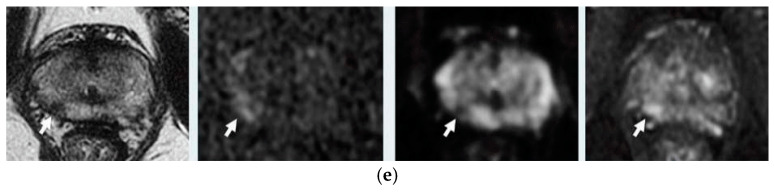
Axial MRI example in post-radiation therapy (RT) by Pecoraro et al. [16]. (a) MR images in 82-year-old man with biochemical recurrence (BCR) (prostate-specific antigen–PSA = 0.09 ng/mL) without suspicious foci of local recurrence, Prostate Imaging for Recurrence Reporting PI-RR 1. (b) MR images in an 85-year-old man with BCR (PSA = 0.09 ng/mL), show diffuse hyperintensity ta high-b value diffusion-weighted imaging DWI (arrows), PI-RR 2. (c) MR images in a 68-year-old man with BCR (PSA = 5.2 ng/mL) indicate hypointense focus at T2weighted Imaging (T2WI) (arrow), low intensity on the apparent diffusion coefficient (ADC) map (arrow) and no hyperintensity at DWI and no early enhancement on dynamic contrast enhancement (DCE), PI-RR 3. (d) MR images in an 83-year-old man with BCR (PSA = 3.1 ng/mL) with similar findings with previous patients and hyperintensity at high-b value DWI and ADC map and not completely matching the enhancement area in DCE, PI-RR 4. (e) MR images in a 73-year-old man with BCR and PSA = 2.4 ng/mL with the same PCa recurrent evidence with PI-RR 4, however, observed matching the enhancement area on DCE image, PI-RR 5.
